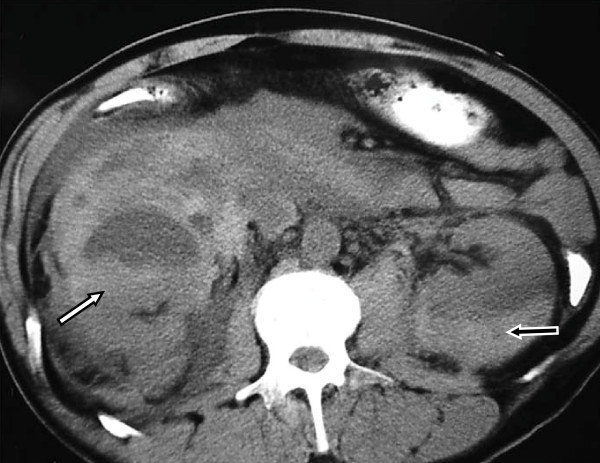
Computed tomography scan demonstrating an extended right retroperitoneal hematoma (white arrow) combined with a left subcapsular renal hematoma (black arrow).
